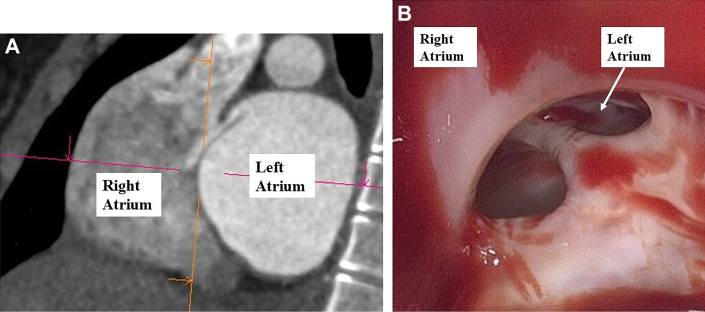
Preoperative and Intraoperative Images of the ASD (A) Preoperative CT showing the ASD. (B) Intraoperative inspection of the right atrium revealed the ASD with fibrous tissue components. ASD = atrial septal defect; CT = computed tomography.
